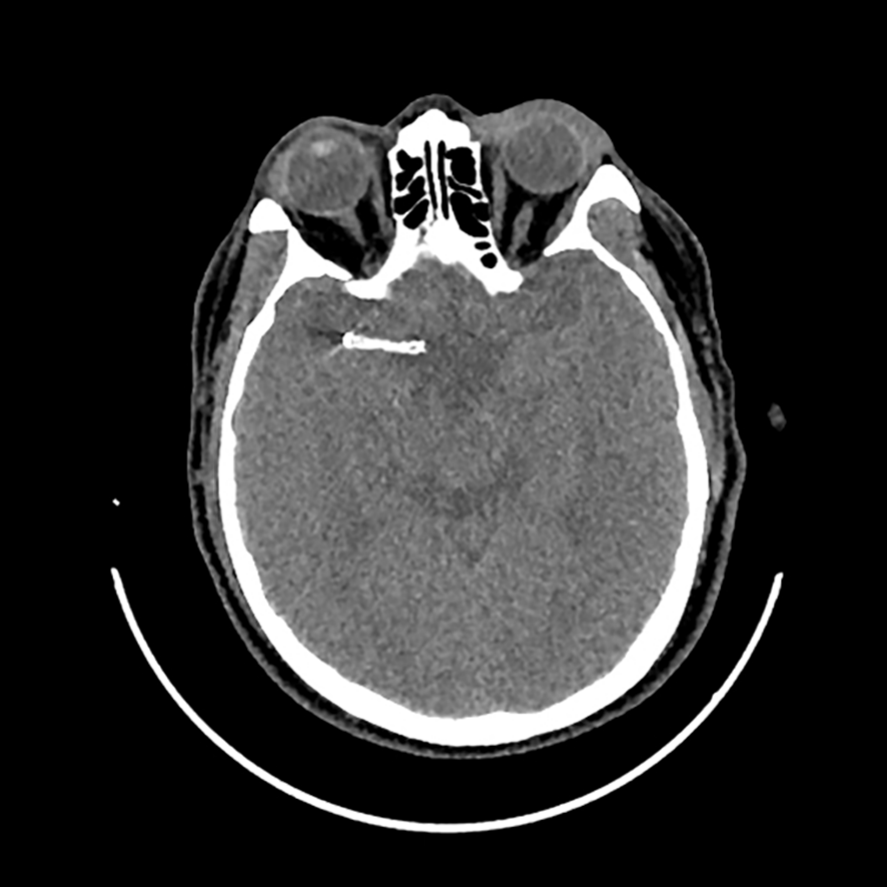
Implantation of stent in his middle cerebral artery in the computed tomography examination.
